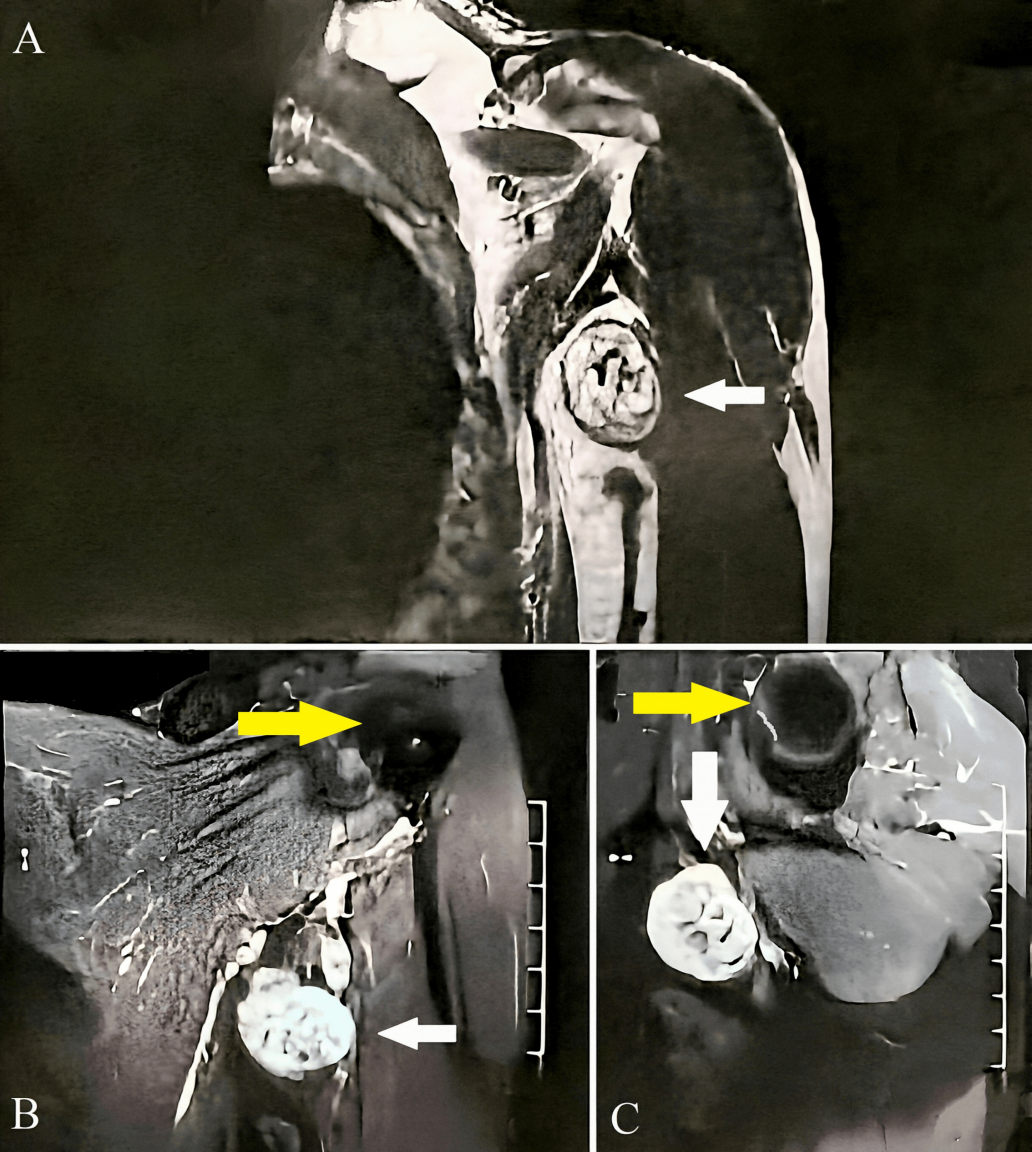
Contrast-enhanced magnetic resonance images of the left shoulder showing a well-encapsulated T1 hypointense, T2 isointense, and STIR hyperintense mass lesion (shown with a white arrow) with heterogeneous enhancement on contrast study involving anteroinferior aspect of the left axilla region inferior to the axillary vessels in coronal view (A on the top and B on the bottom left) and axial view (C on the bottom right). Head of the humerus shown with a yellow arrow STIR: Short-TI inversion recovery
